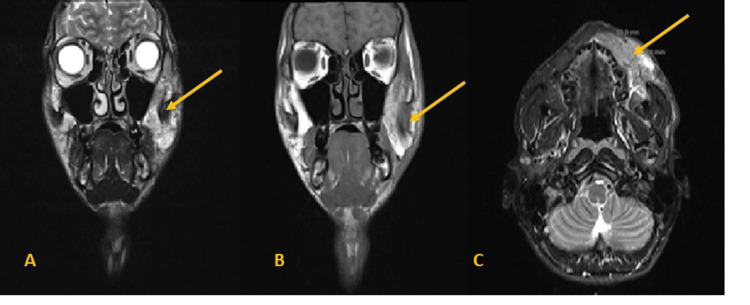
MRI of the face A: T2 weighting with fat saturation, B: T1 weighting after contrast, C: T2 weighting
